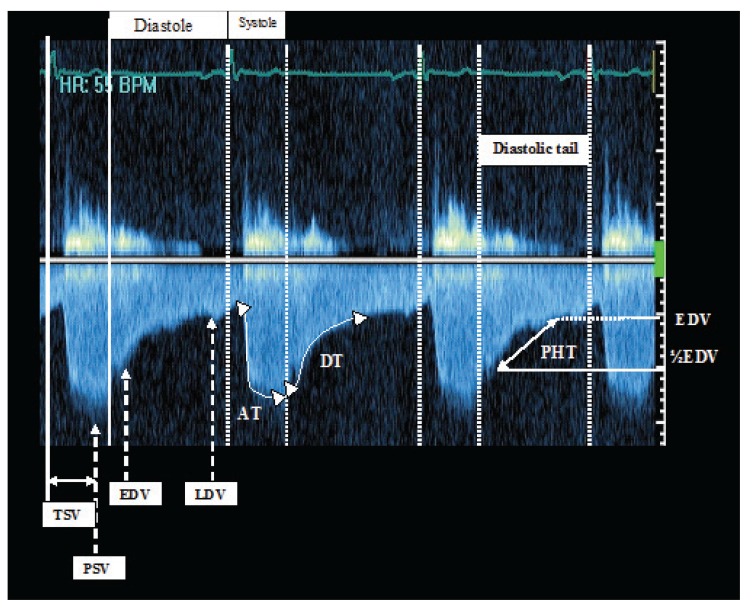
Continous-wave Doppler echocardiography of descending aorta. AT : systolic acceleration time, DT: deceleration time, EDV: early diastolic velocity, LDV: late diastolic velocity, PHT: pressure half-time (of diastole), PSV: peak systolic velocity, TSV: time to peak systolic velocity.
